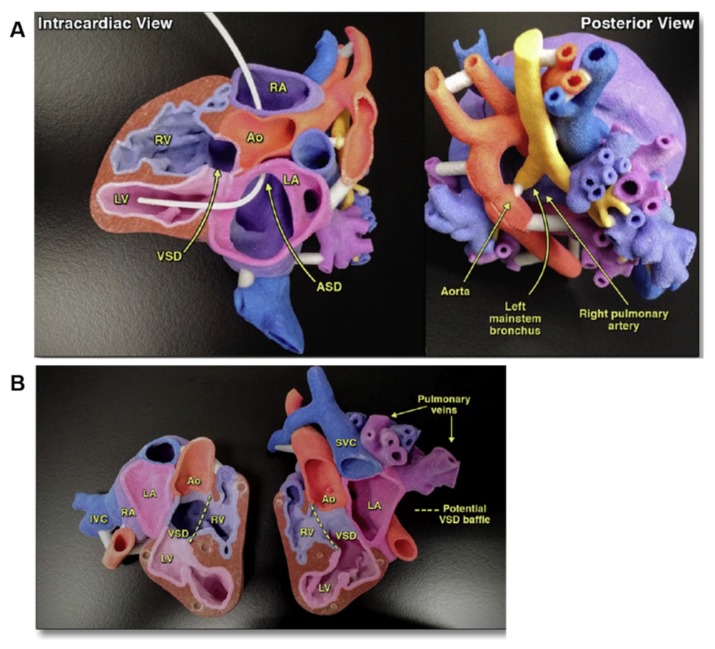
(A) 3D model printed to visualize the complex anatomy and aid surgical planning of a four-month-old patient with superoinferior ventricles, atrial septal defect (ASD), ventricular septal defect (VSD), left lung collapse, leftward shift of the heart, and compression of the left mainstem bronchus. (B) A 3D model obtained from cardiac MRI of an eight-month-old patient with situs inversus, dextrocardia, double-outlet right ventricle (DORV), and L-malposed great arteries was printed to guide next steps in surgical management. Reprinted with permission from Anwar et al. [39]. Copyright © 2020 Elsevier.
